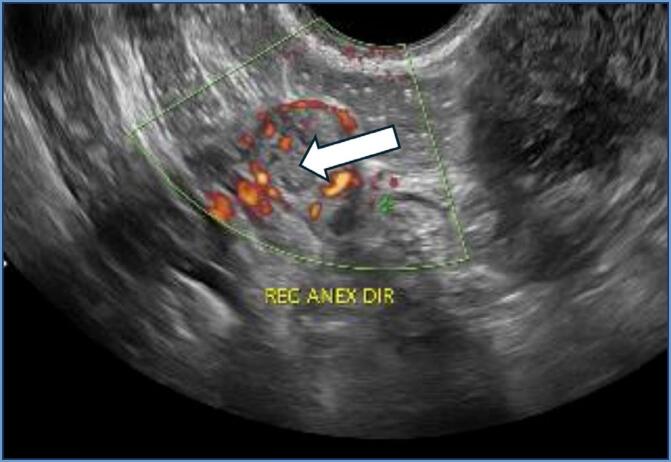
Transvaginal ultrasound from the study cohort, showing a right adnexal mass with peripheral vascularization (arrow), consisent with the classical tubal ring sign of ectopic pregnancy
